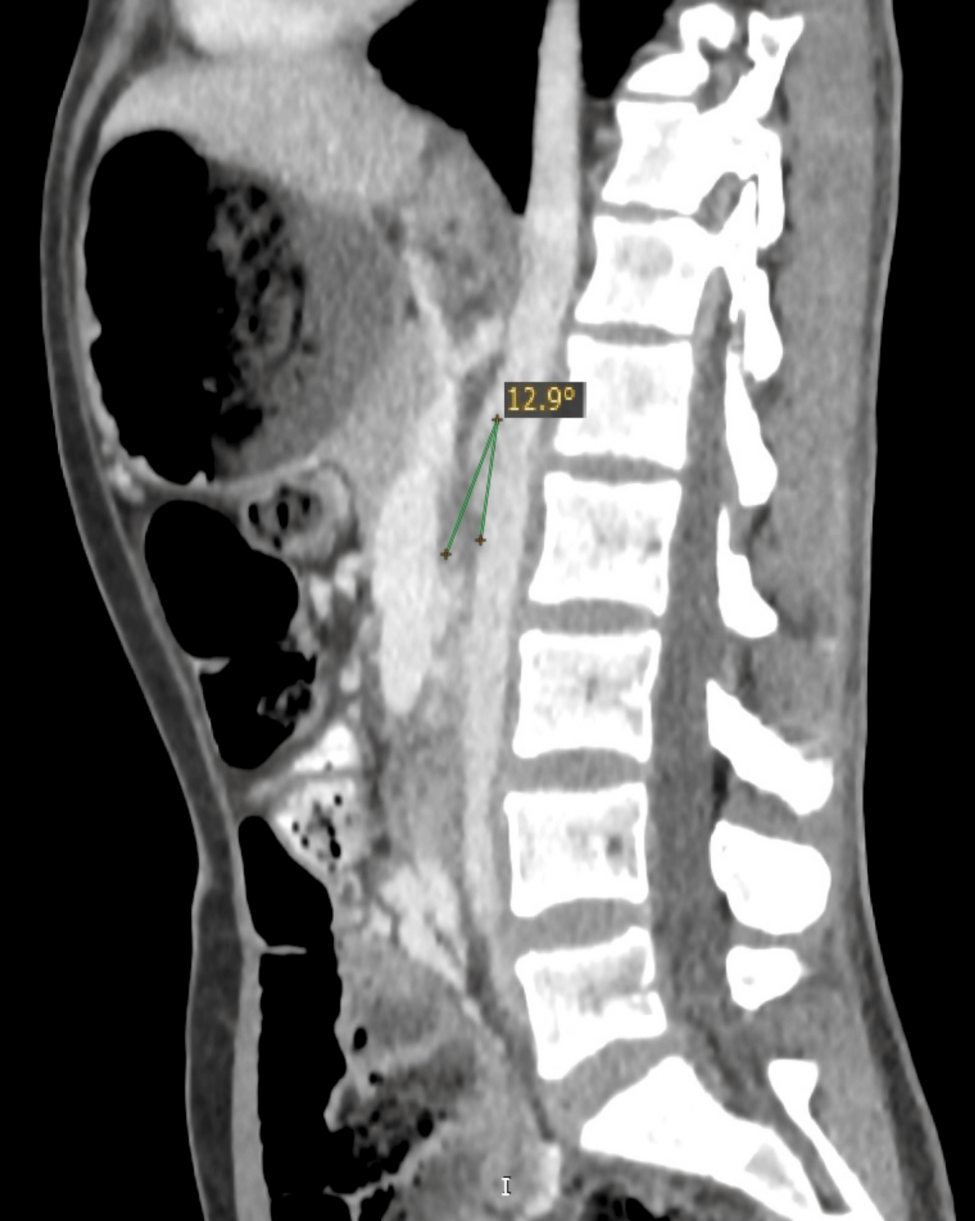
Sagittal plane intravenous contrast-enhanced computed tomography The angle of the aortomesenteric confluence is measured at 12.9°, while normal values of the angle range between 38° and 65°, indicative of SMA syndrome SMA: superior mesenteric artery
